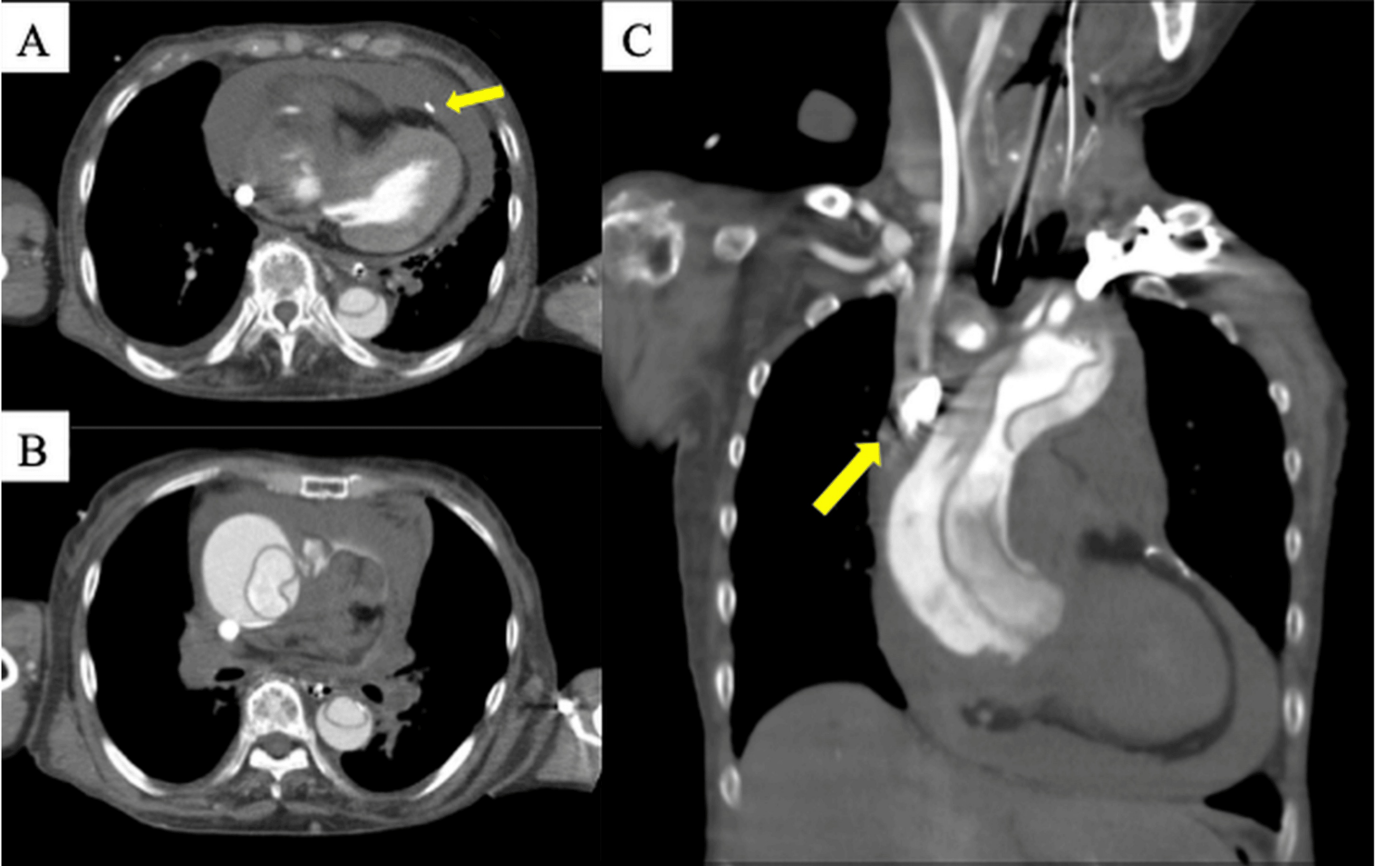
Contrast-enhanced computed tomography (CT) images. (A) Axial image at the right atrial level showing compression of the right atrium, with the drainage cannula positioned by the surrounding hematoma. The arrow indicates the pericardial drain. (B) Coronal image showing intimal rupture (entry) of the ascending aorta. (C) Axial image at the level of the superior vena cava (SVC) showing the drainage cannula advanced toward the SVC. The arrow indicates the tip of the ECMO drainage cannula in the SVC.
